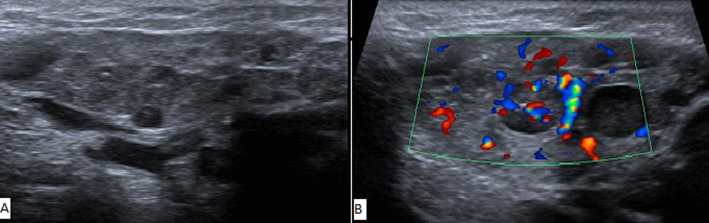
ultrasonography images A) presence of multiple hypoechogenic areas within the parenchyma; B) hypervascularization of the gland parenchyma
